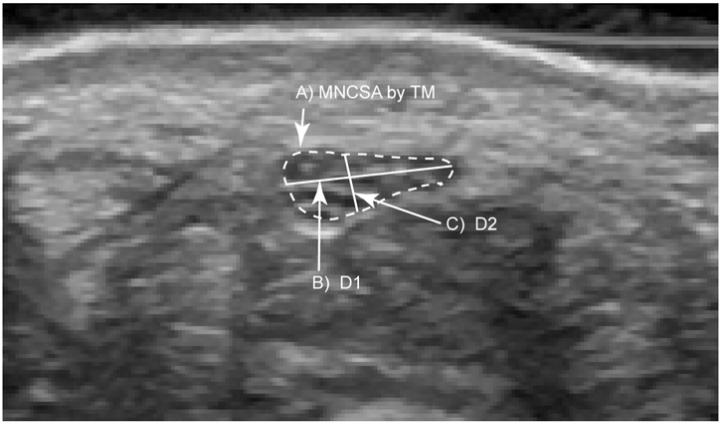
Ultrasound image of the median nerve at the proximal carpal tunnel. A) median nerve cross-sectional area by tracing method; B) longitudinal diameter; C) vertical diameter.
